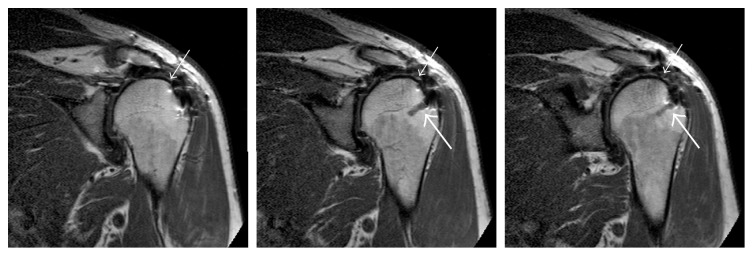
A series of adjacent coronal MR images taken at 18 months after surgery showing the graft (thin white arrow) and suture anchor (thicker white arrow). Mild arthritic changes are also seen.
